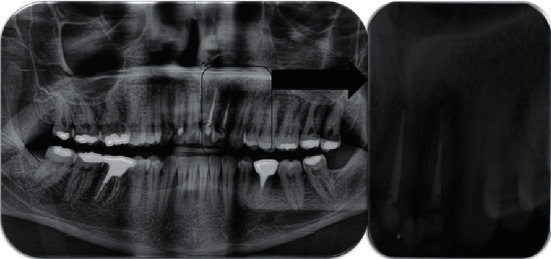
Initial panoramic and periapical radiography of the periapical lesion of tooth #22.
